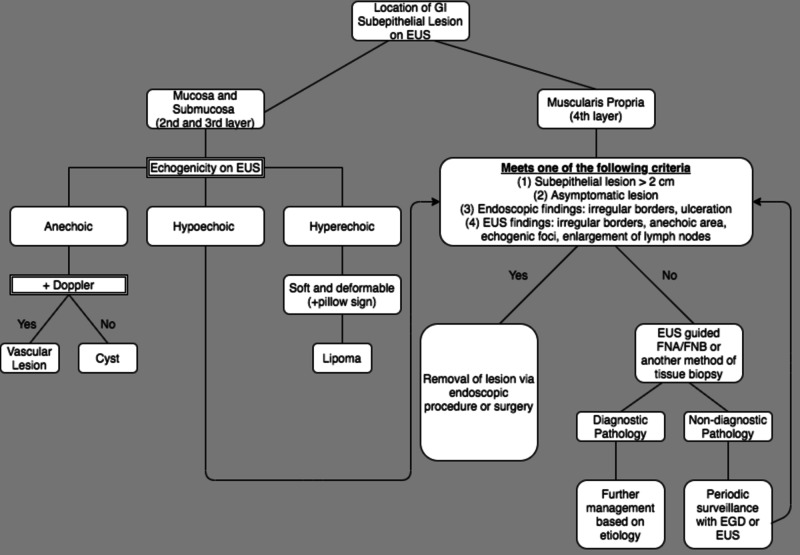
Management for gastrointestinal subepithelial lesions GI: gastrointestinal, EUS: endoscopic ultrasonography, EGD: esophagogastroduodenoscopy, FNA: fine-needle aspiration, FNB: fine-needle biopsy.
